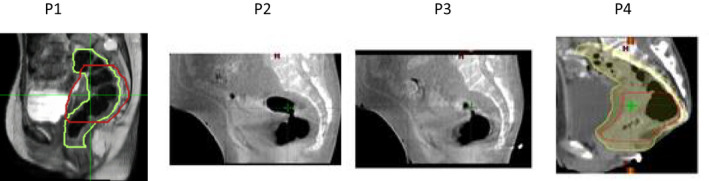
Clinical examples of typical air cavity size seen on the MR (P1) and CBCTs of four different patients.
